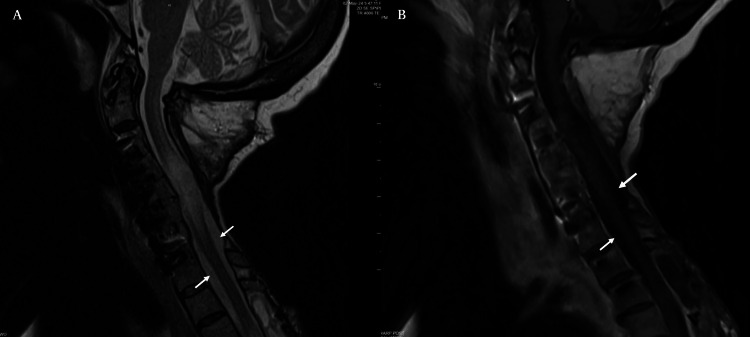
Nine-month post-operative MRI Follow-up MRI of the cervical spine at nine months post-operatively, further demonstrating significant resolution of mass lesions on T2 (A) and post-contrast T1 (B) imaging, with arrows indicating areas of even greater CSF signal, free from prior visualized compressive lesions.
